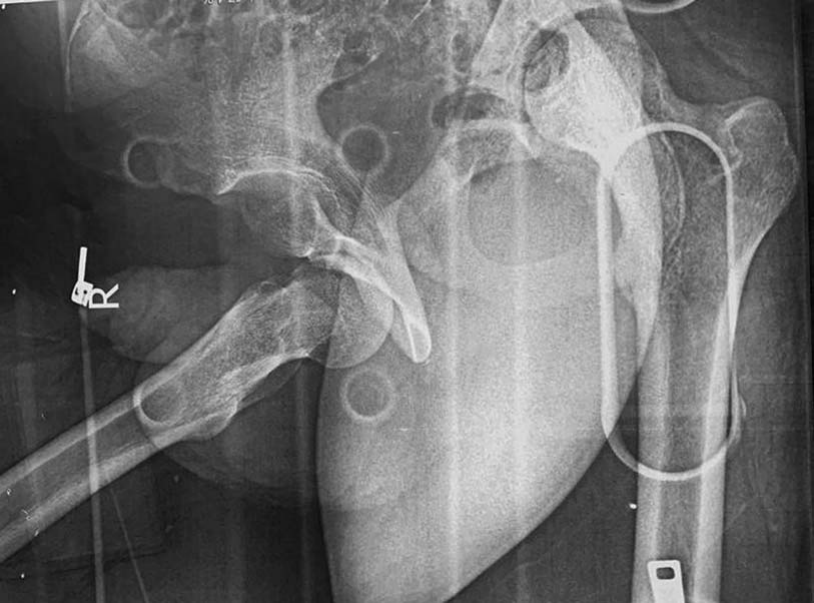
A pelvic obturator Judet view X-ray. An inferior dislocation is seen in the right hip without any sign of fracture.
